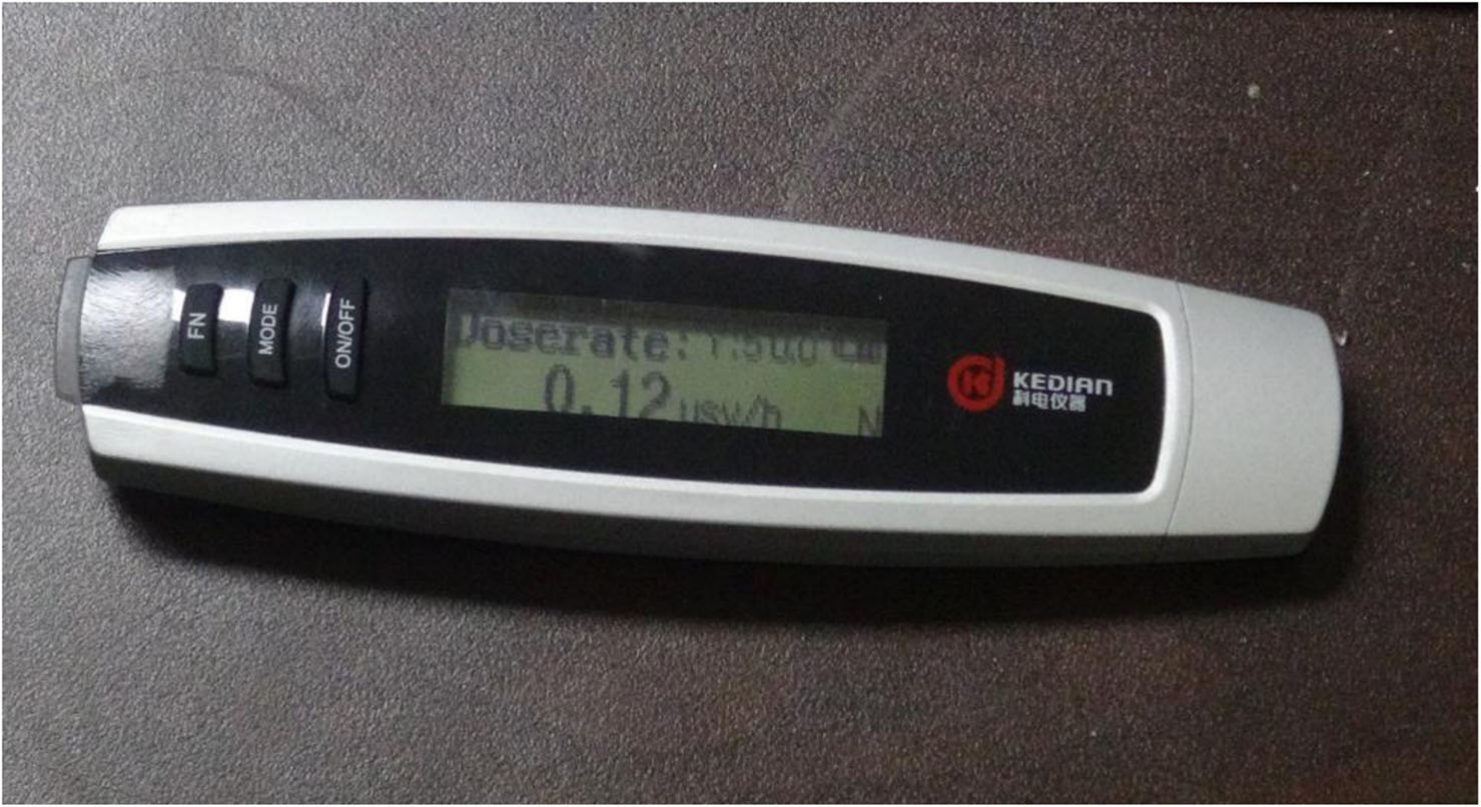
X-ray dosimeter Ray 3000. It measures the radiation dose rates in millisievert per hour and microsievert per hour
